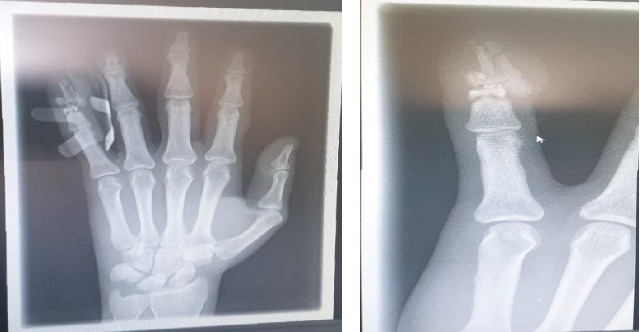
X-ray hand left findings: (a) finer bony details are obscured by the plaster slab and (b) an internal fixation pin is noted in the left little finger for fractures of the middle and distal phalanges.
